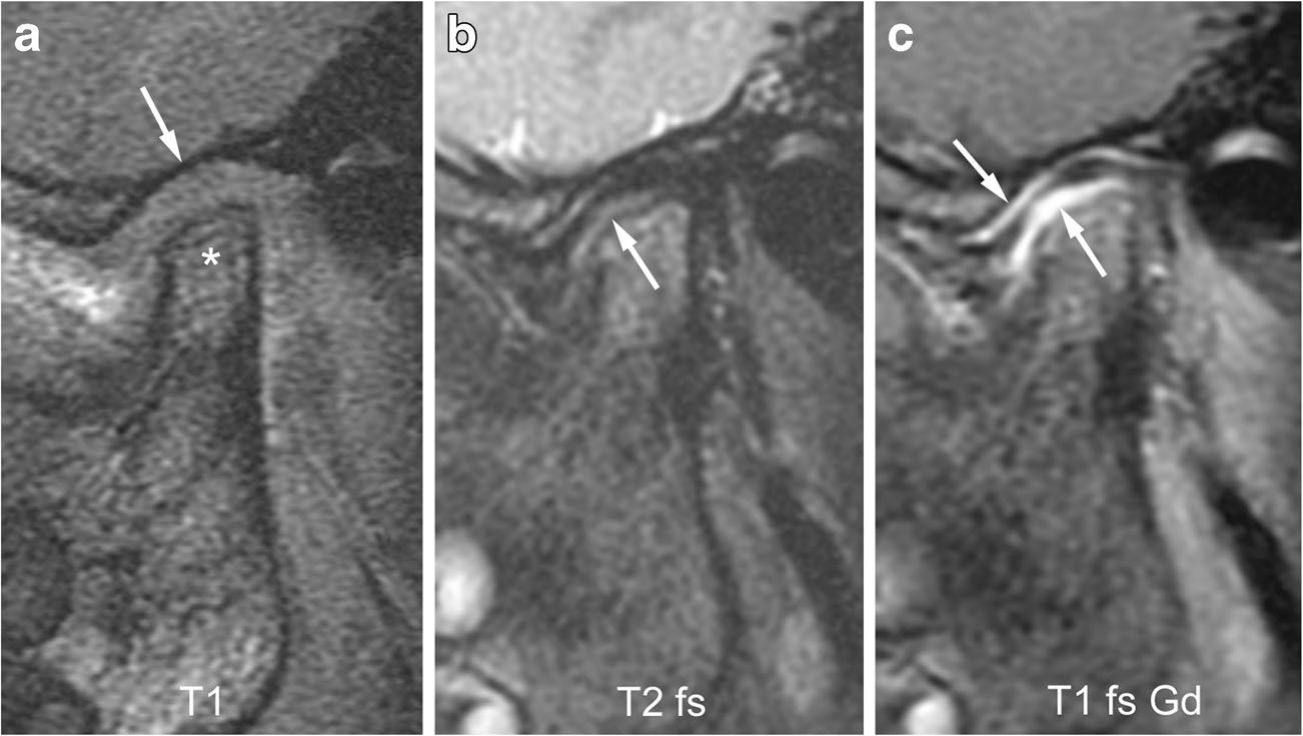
Mild synovial thickening (grade 1) and severe joint enhancement (grade 2) on sagittal oblique images in a 5-year-old boy. a There moderate flattening of condyle (*) and mild flattening of temporal bone (arrow) on T1-weighted image (TR/TE 300/4.2 ms, flip angle 80°, without fat saturation). b Fluid-sensitive, fat-saturated T2-weighted fast spin echo image (TR/TE 5,400/77 ms) shows tissue with intermediate signal intensity exceeding 1-mm width in lower joint space (arrow). c Postcontrast fat-saturated T1-weighted image (TR/TE 670/10 ms) shows high signal intensity (arrows) involving both the upper and lower joint compartments. T1-weighted, T2 T2-weighted, T2 fs fat saturated T2-weighted
